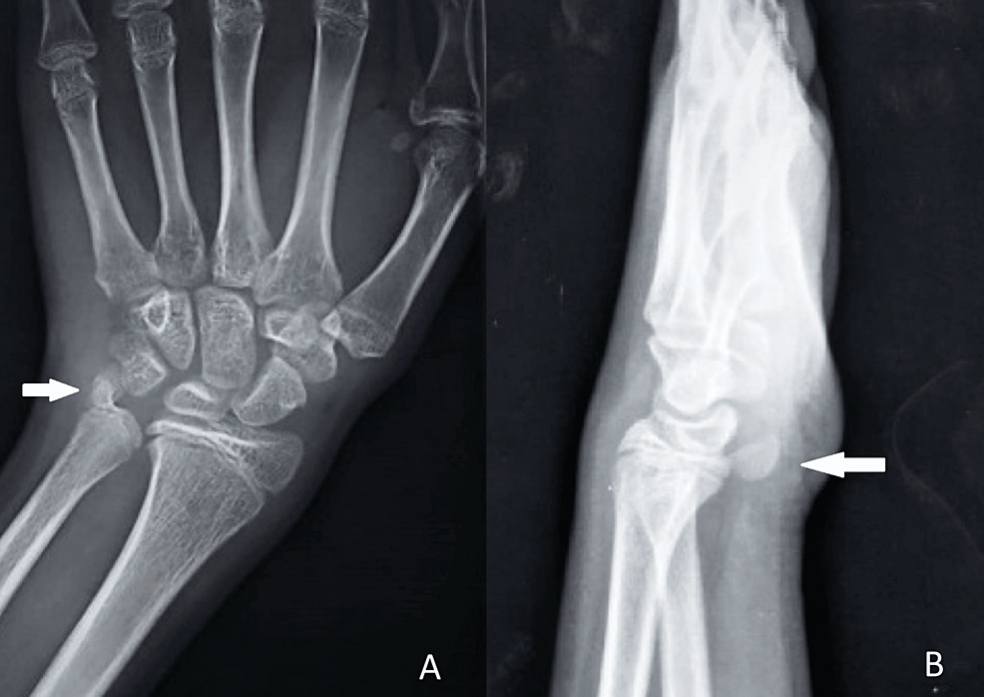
Plain radiographs showing the pisiform bone (white arrow) lying close to the ulnar styloid on (A) AP views and just anterior to the lunate on (B) lateral views. AP, anteroposterior
